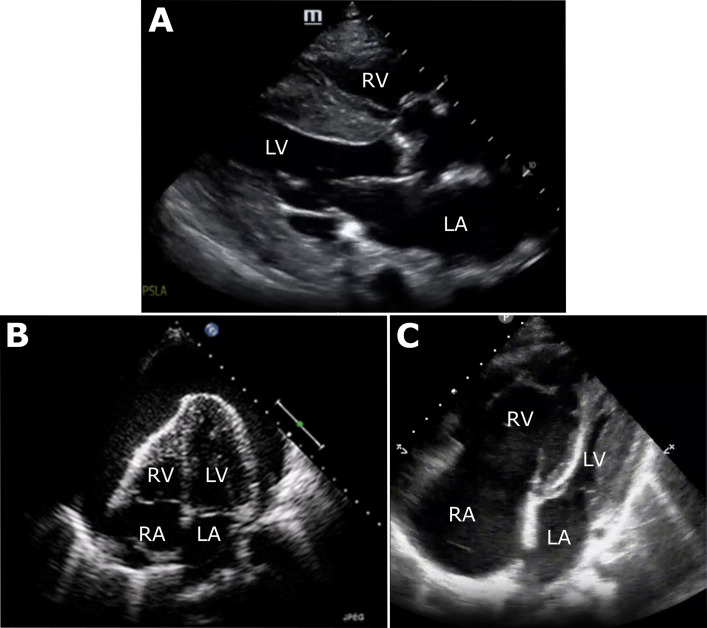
Focused cardiac ultrasound (FOCUS) findings which suggest congestive heart failure and volume overload. A Parasternal long-axis view demonstrating concentric left ventricular (LV) hypertrophy, which can be seen in heart failure with preserved ejection fraction. B A large pericardial effusion with evidence of right ventricular (RV) collapse, which may be seen in cardiac tamponade. Pericardial effusions can result from volume overload as well as numerous other etiologies. C Severe right atrial (RA) and RV enlargement, which can be seen in right-sided heart failure, typically resulting from pulmonary pathology. LA, left atrium
